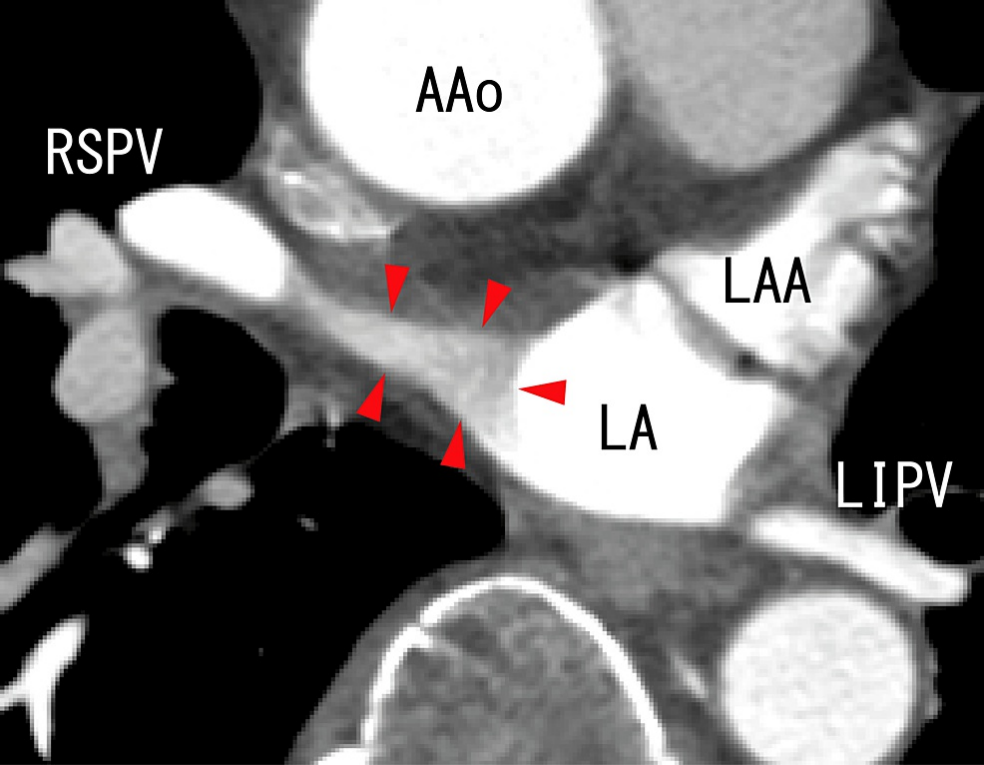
Axial images from an 80-MDCT scan obtained after one month of standard-dose heparin-warfarin remedy showing LA thrombi Axial images from an 80-MDCT scan obtained after one month of standard-dose heparin-warfarin remedy showing the RSPV, left inferior pulmonary vein (LIPV) and LA. There were no images of thrombi in the RSPV, suggesting that the thrombi in the RSPV had resolved. The dark area of the LA became slightly larger (arrowheads), suggesting that the thrombi might have become larger. However, the observed range of the LA was not necessarily the same between Figure 2 and Figure 5. AAo, ascending aorta; LA, left atrium; LAA, left atrium appendage; RSPV, right superior pulmonary vein; 80-MDCT, 80-slice multidetector computed tomography
